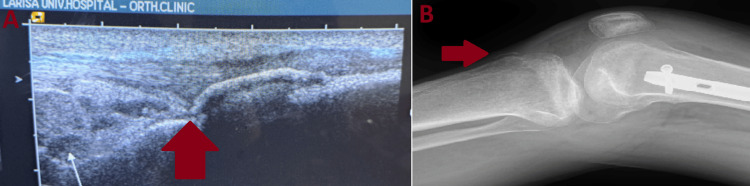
Ultrasound and X-ray imaging of the injured knee after the third traumatic incident of the patient A bony avulsion can be seen in both images A) Ultrasound image of the injured knee in longitudinal view. A partial rupture of the patellar tendon and a bone avulsion can be seen near the tibial tubercle. B) Lateral X-ray of the injured knee
